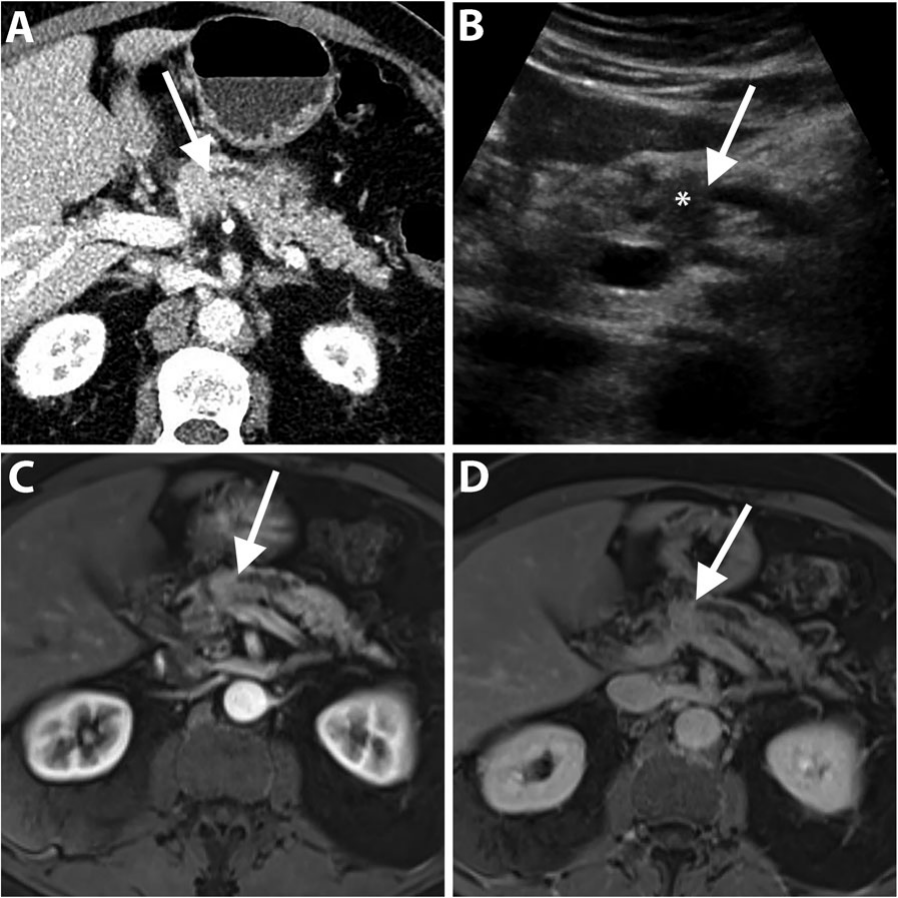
A 72-year-old man with isoattenuating PDAC. Axial pancreatic phase CT image (a) shows MPD stricture (arrow) at the pancreatic body without visible mass. Transverse transabdominal ultrasound image (b) shows a hypoechoic mass (asterisk) at the site of MPD stricture (arrow). Dynamic contrast-enhanced MR images using fat-suppressed T1-weighted sequence in the arterial (c) and portal venous (d) phases show MPD stricture (arrow) but fail to demonstrate a distinct mass. Subsequent pancreaticoduodenectomy revealed PDAC
